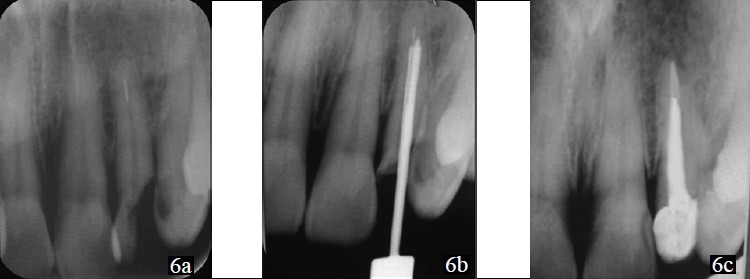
(a) – Radiograph showing the separated file; (b) – Extractor with plunger sleeving and gripping the fragment; (c) – Post-endodontic restoration given
